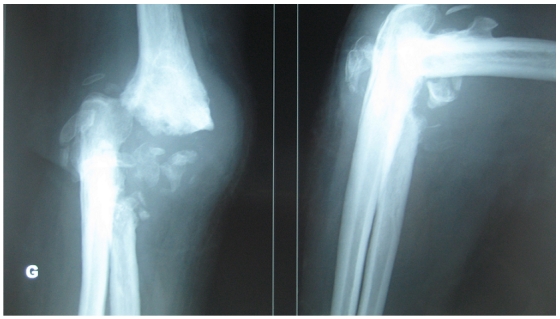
Radiographies de face et de profil du coude montant les séquelles post infectieuses
